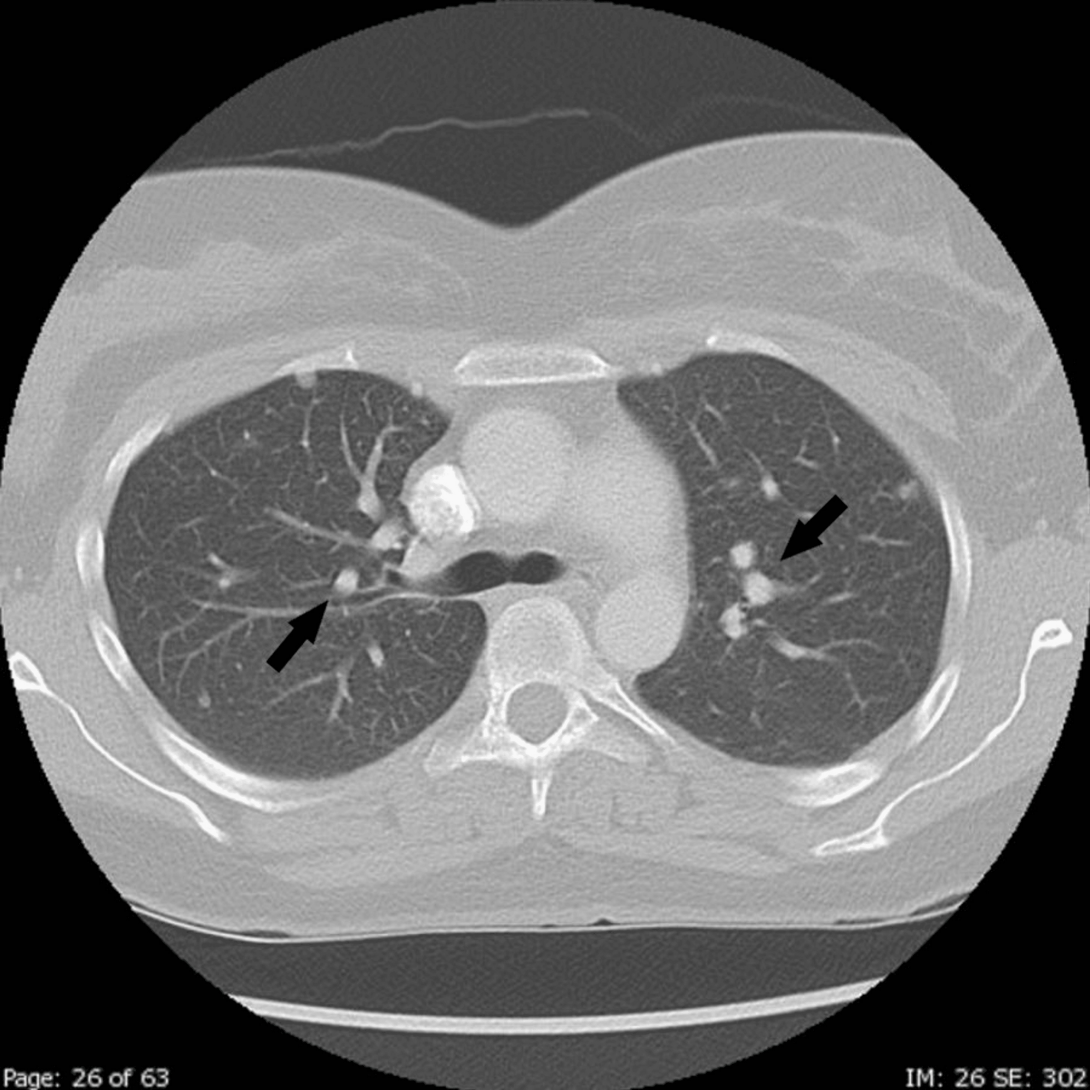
CT showing multiple metastatic lung nodules (black arrows)
